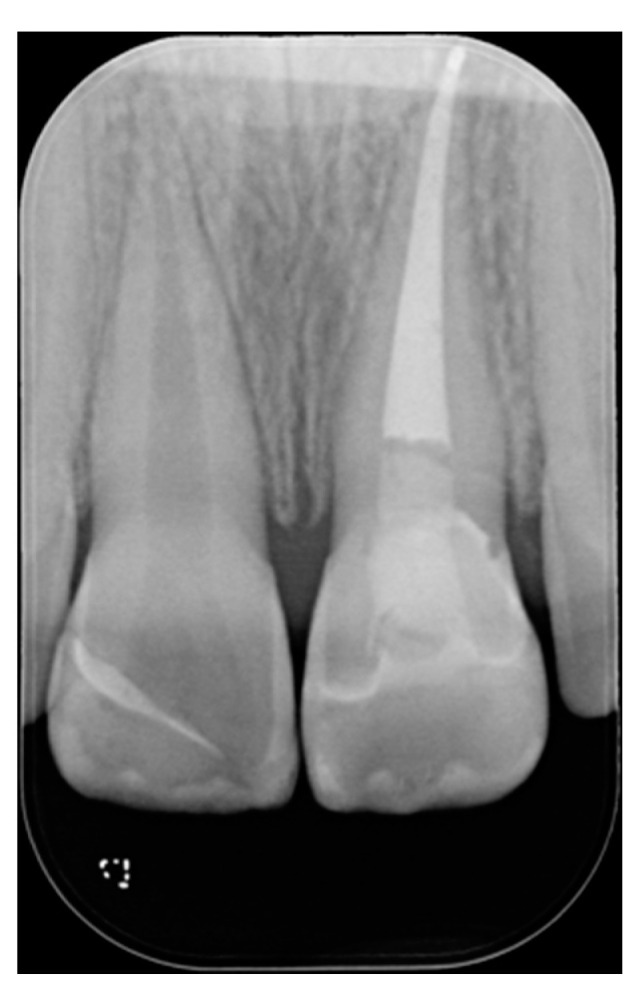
At 1.5 years, post-operative radiographic view. Reprinted from Restauri diretti nei settori anteriori, G. Paolone, S. Scolavino, © 2021, with permission from Quintessence Publishing Italy.
